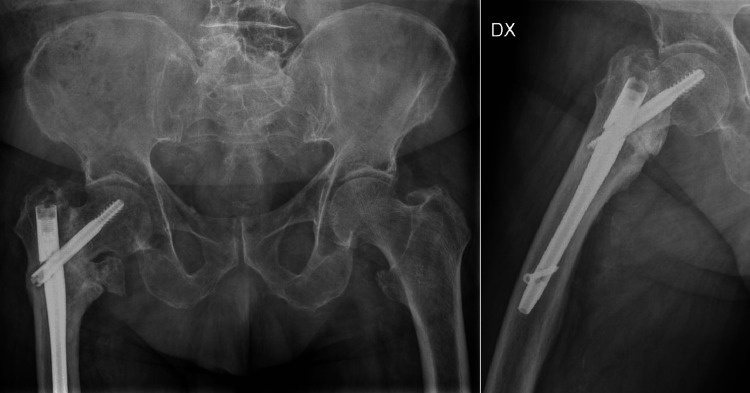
Plain antero-posterior radiographs showing nonunion of a pertrochanteric fracture with fatigue failure and breakage of the Gamma cephalomedullary nail
